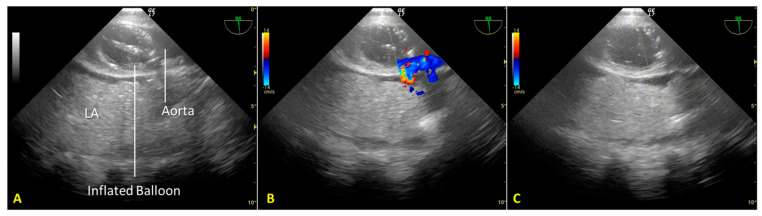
(A). Mid-esophageal descending aortic long-axis view scan: the endovascular occluder (RELIANT^®^ catheter balloon, Tamworth, UK) is visible in the descending thoracic aorta. (B). Incomplete occlusion of the thoracic aorta during the initial phase of regional extracorporeal support for organ retrieval. Transesophageal color Doppler echocardiography documents the passage of blood around the aortic occluder. (C). Complete occlusion of the thoracic aorta after additional filling of the RELIANT^®^ AB. Absence of blood flow in the thoracic aorta above the occluder. LA—lung atelectasis.
